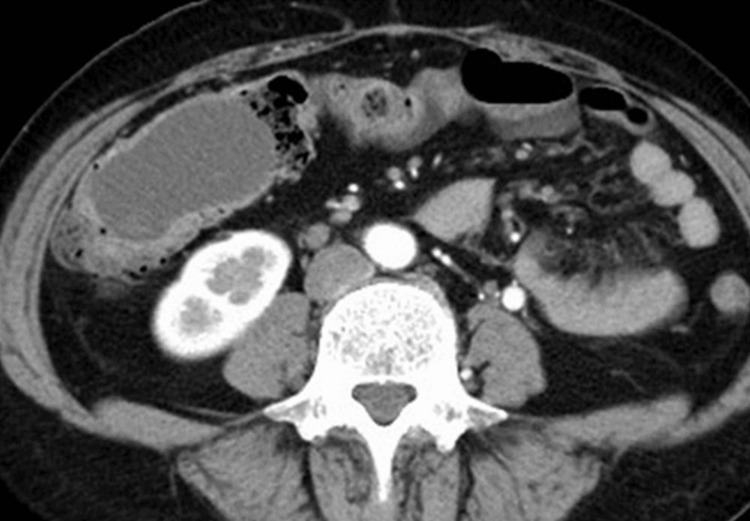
Contrast-enhanced CT image of a cystic mass The leading point of the colonic invagination was a cystic mass with a diameter of 50 mm. The mass was homogenous and had no solid components.
